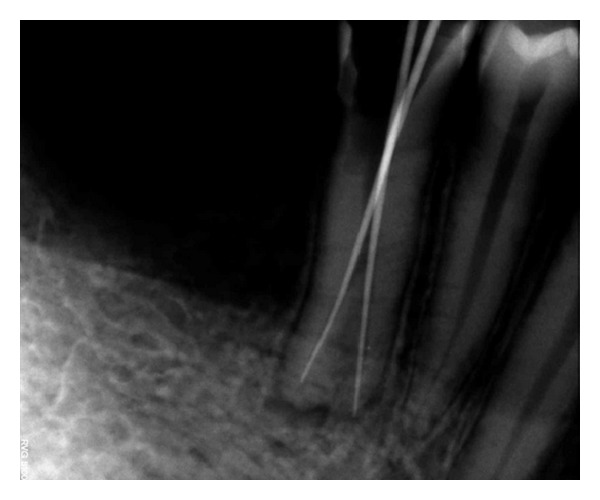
Periapical radiograph showing the working length.
